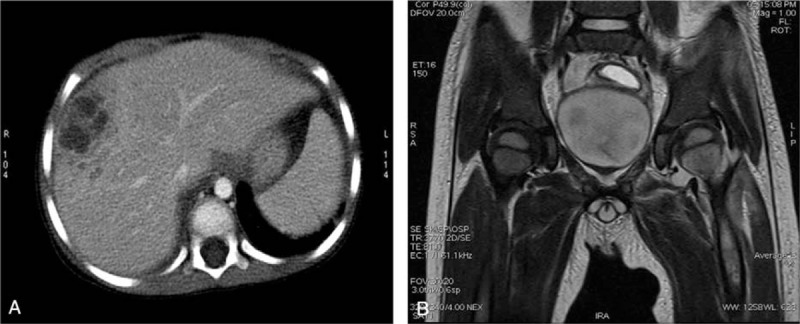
(A) Contrast-enhanced computed tomography showed multiple hepatic abscesses. (B) Magnetic resonance imaging revealed inflammation of soft tissue around left hip joints.
